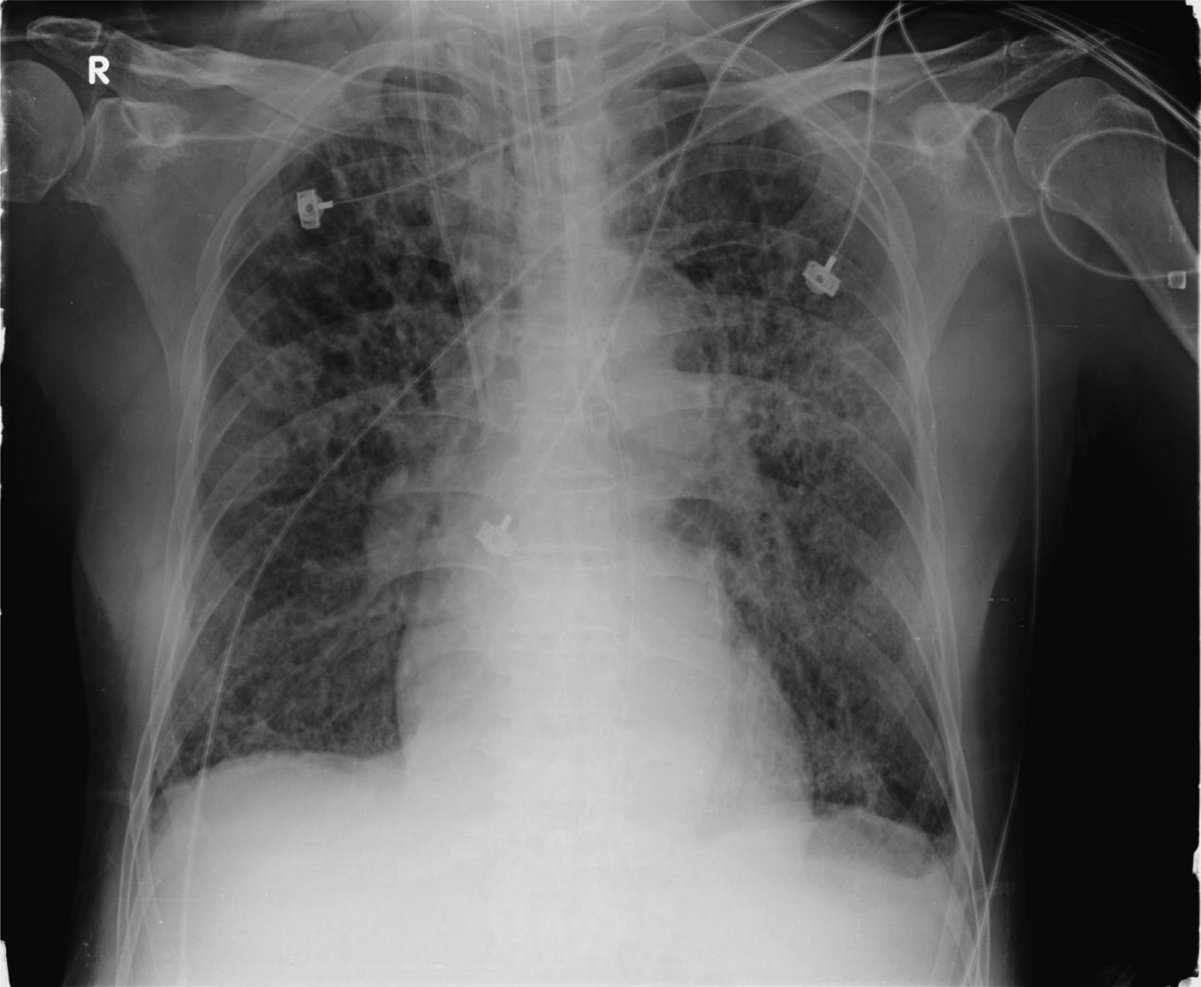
Typical radiological findings seen on a chest radiograph of the patient following endotracheal intubation for acute respiratory distress syndrome.
